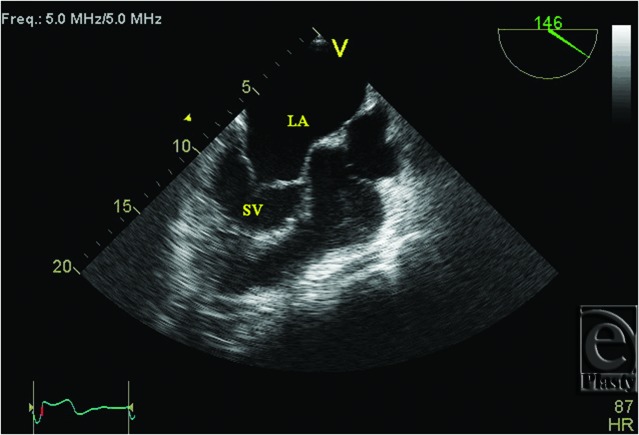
Transesophageal echocardiogram showing hypertrophied SV and dilated LA. LA indicates left atrium; SV, systemic ventricle.
